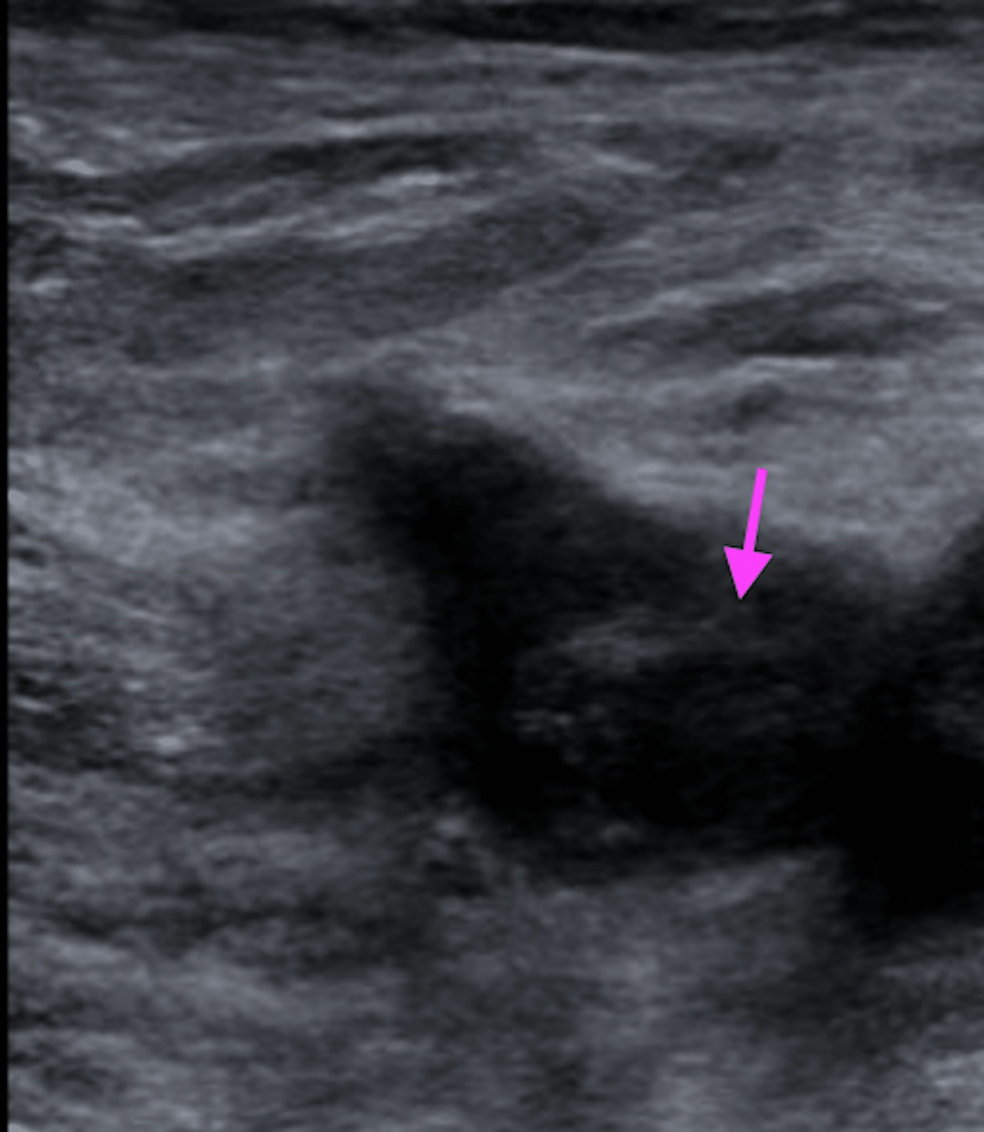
Nonocclusive DVT involving the left common femoral vein seen on ultrasound in Case 2 DVT: deep vein thrombosis
